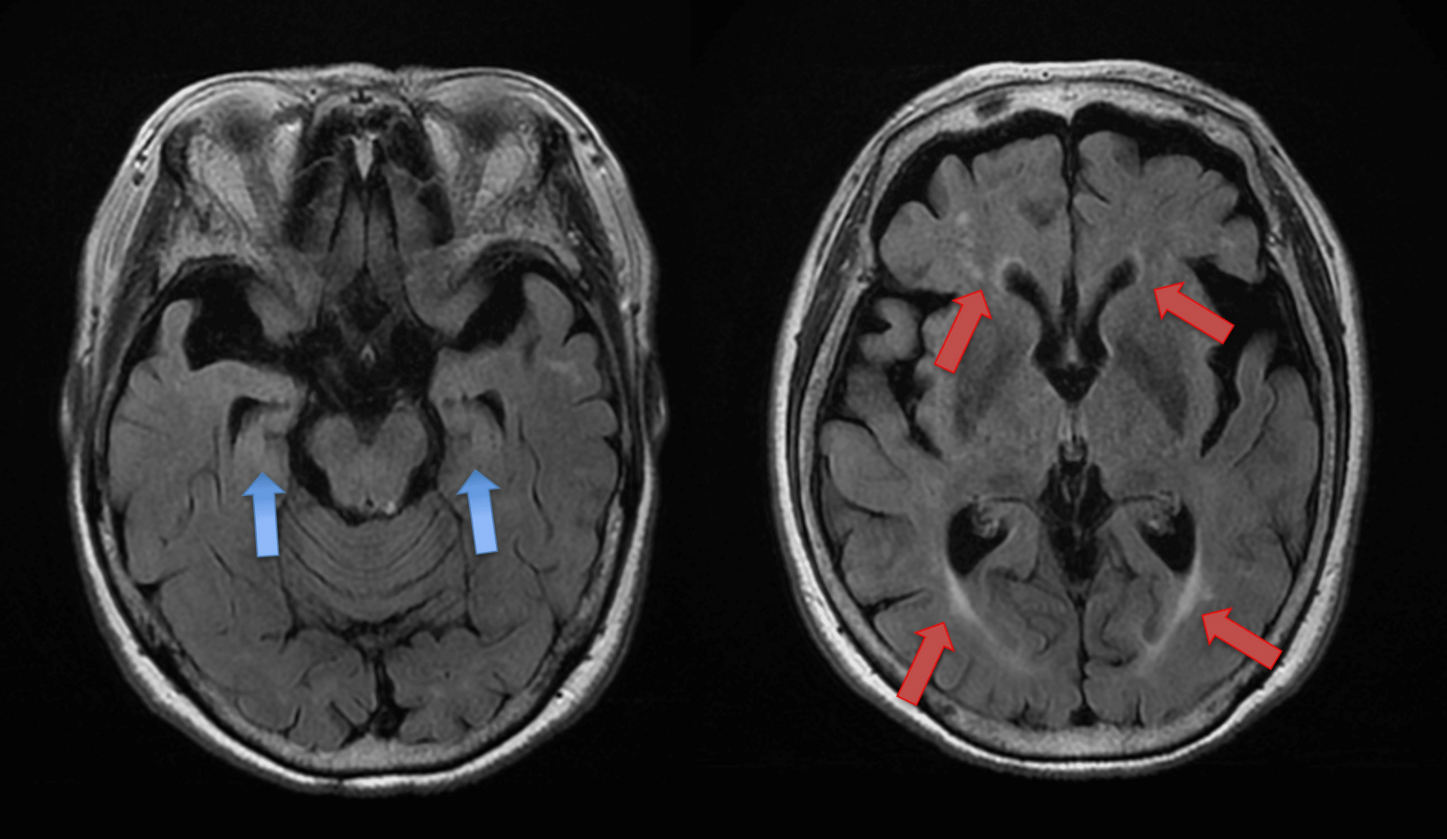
Pre-treatment brain magnetic resonance imaging (MRI) findings Axial T2-weighted fluid-attenuated inversion recovery (T2-FLAIR) magnetic resonance imaging (MRI), obtained prior to initiation of lecanemab treatment, reveals no hemorrhagic lesions or vascular edema, mild atrophy of the medial temporal lobes (blue arrows) and relatively prominent chronic ischemic changes in the periventricular and deep white matter (red arrows).
